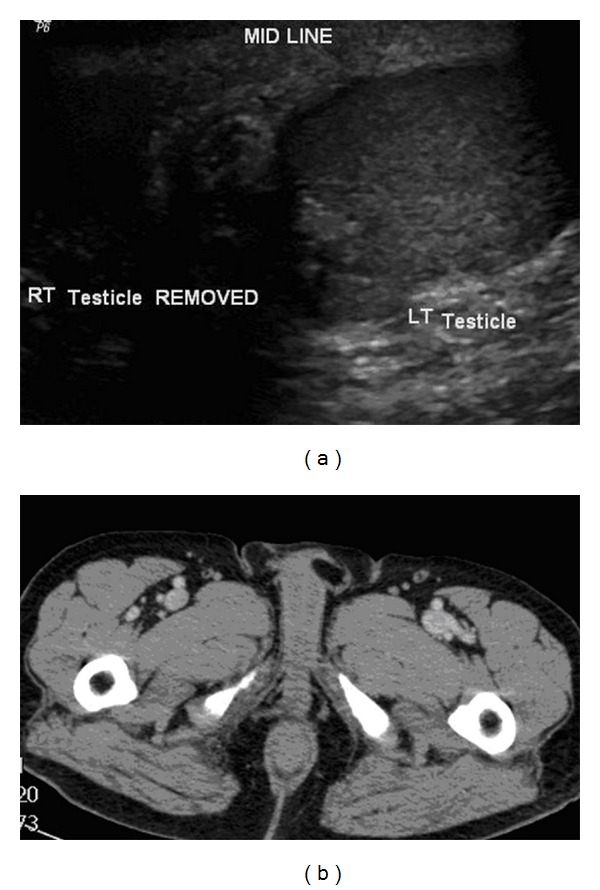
Follow up US and CT scans (a and b) showing no evidence of local recurrence.
